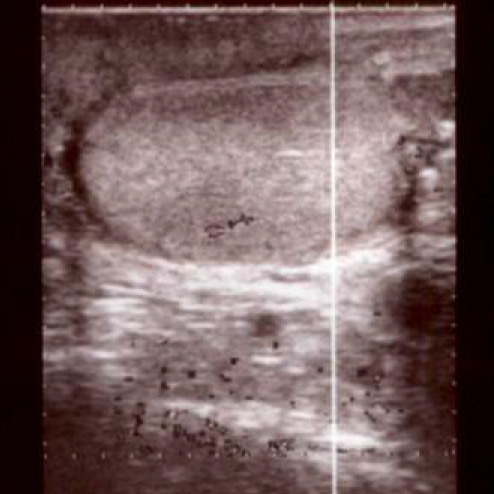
Ultrasonography after 3 months.
